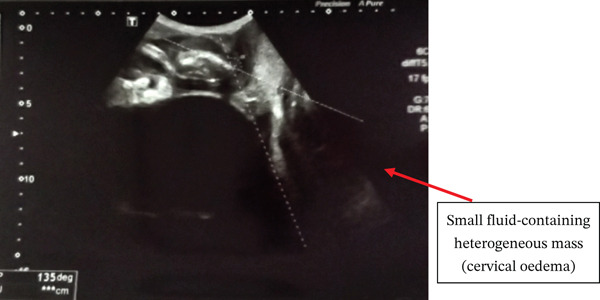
Intrapartum ultrasound showing fetal head engagement.
